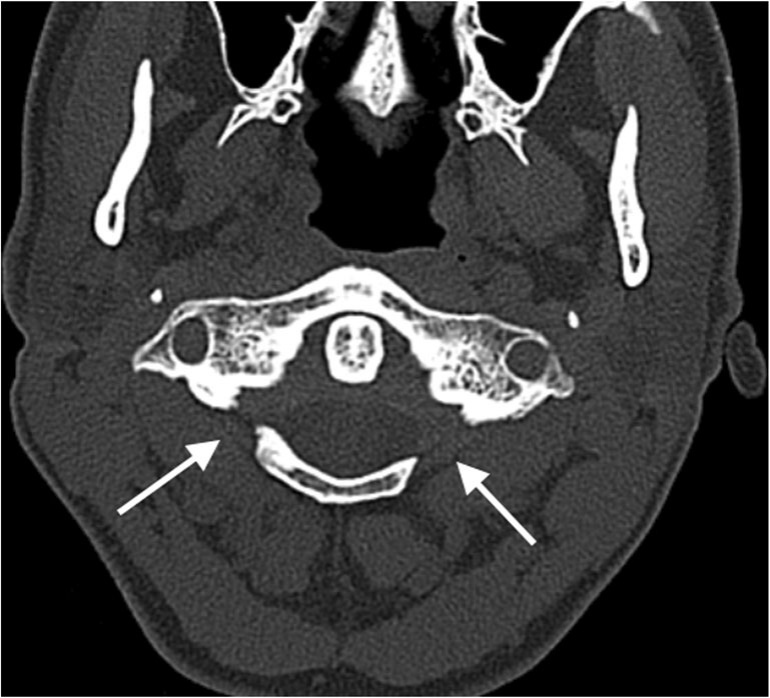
Bilateral posterolateral rachischisis: axial CT image through the atlas (C1 vertebra) of the craniocervical junction of a 47-year-old female who suffered blunt trauma to the head and complained of neck pain. Bilateral developmental posterolateral rachischisis is evident (*arrows*). There was no acute fracture of the craniocervical junction present
